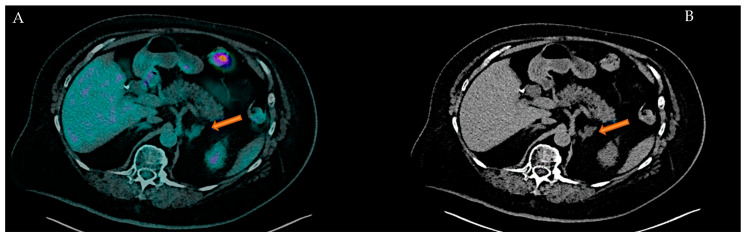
56-year-old male with history of non-small cell lung cancer (NSCLC) and a left adrenal non-secreting-mass indicated by arrows. (A): Axial [^18^F]FDG PET/CT fusion imaging shows a focal FDG uptake in left adrenal gland (SUV max: 2.26); (B): Axial CT low-dose imaging shows a low-density (UH −15) nodular lesion (maximum transverse diameter: 8 mm) in the left adrenal gland.
